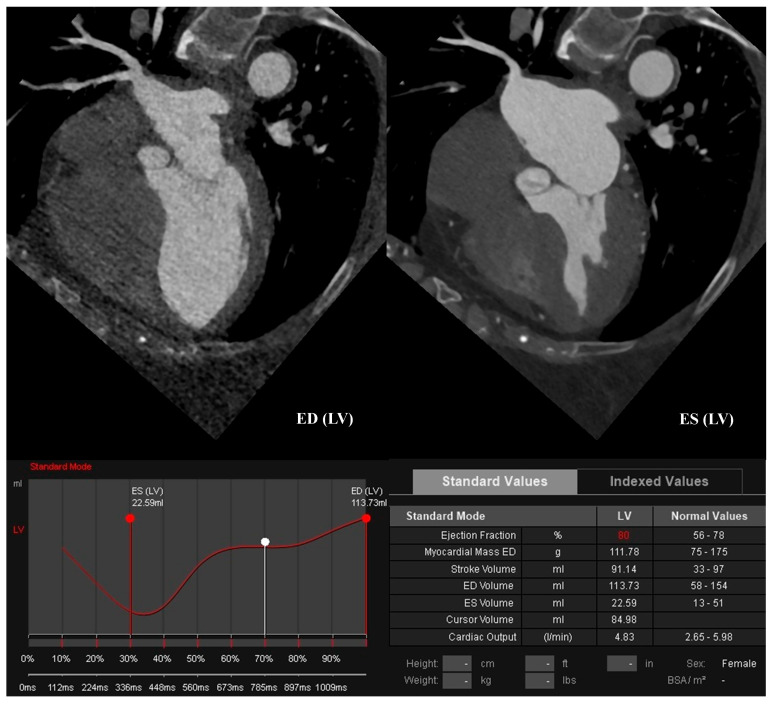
Cardiac computed tomography angiography. Angiographic phase. Left ventricular function assessment—normal left ventricular ejection fraction. ED—end-diastolic, ES—end-systolic, LV—left ventricle.
